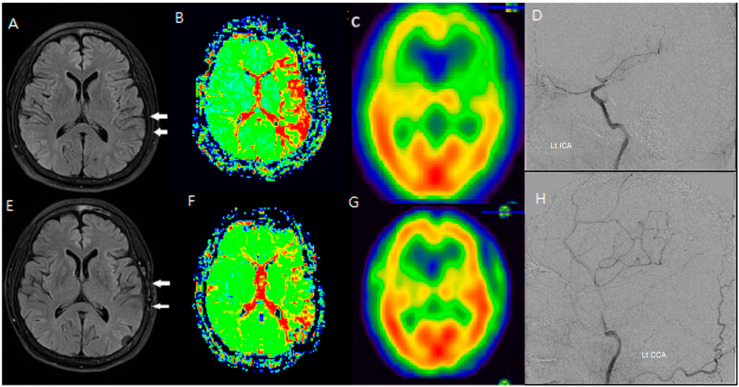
An F/66 patient with left distal ICA severe stenosis. (A–D) Preoperative MR FLAIR, perfusion MR, and SPECT images show prominent hyperintense vessels (white arrows) in cortical regions with a reduced CVR. (E–H) Postoperative imaging reveals the disappearance of HV and improvement in cerebral perfusion, indicating successful revascularization via STA-MCA bypass. HV regression corresponded to an increased CVR and reduced perfusion delay, reinforcing the functional significance of FLAIR findings.
